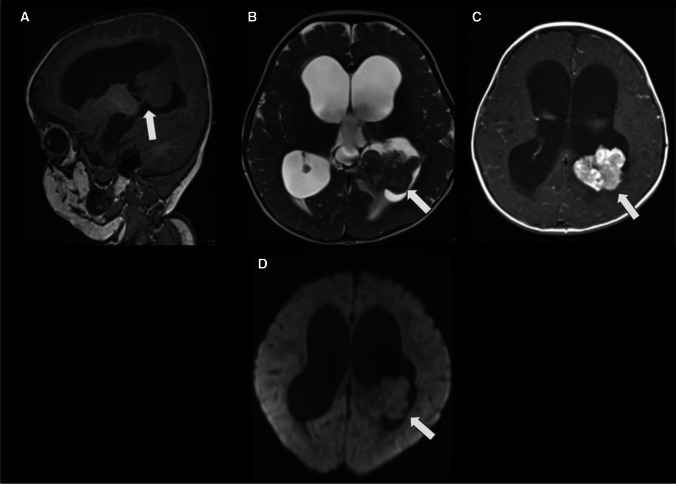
Atypical choroid plexus papilloma (whiter arrows) in an 18-month-old girl with headaches and recent seizures. A A sagittal T1-weighted image shows a lobulated, hypointense mass with suggestion of brain invasion and moderate hydrocephalus. B An axial T2-weighted image reveals that the lesion is predominantly hypointense, with peripheral increased vascularity. In addition, there are signs of brain invasion and a moderate degree of surrounding vasogenic oedema. C An axial contrast-enhanced T1-weighted image demonstrates marked and heterogeneous enhancement of the lesion. D An axial diffusion-weighted image indicates that the mass is isointense in comparison with the adjacent brain
